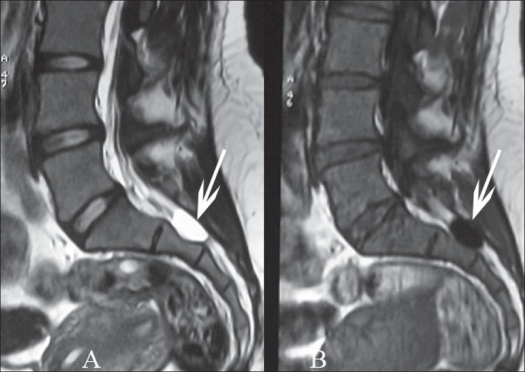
MRI mid segittal T_2_WI (A) and T_1_WI (B) imaging showing a fluid-filled cystic lesion, measuring 2cm in diameter, sitting opposite the second sacral vertebra
